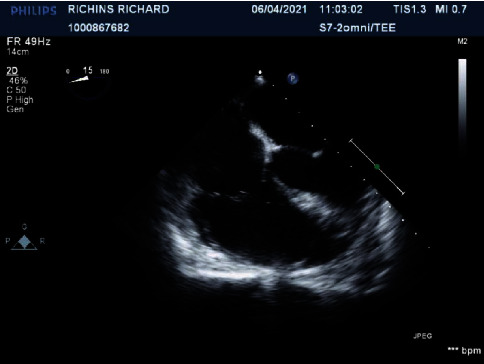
Transesophageal echocardiogram results.
